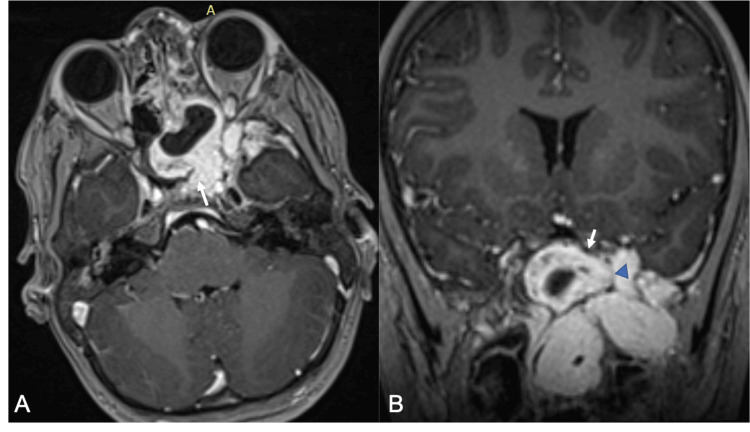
Post-contrast imaging highlighting superior extension with erosion of the sphenoid sinus and intracranial extension. Axial (A) and coronal (B) post gadolinium T1-weighted images showing superior tumor spread beyond the sphenoid sinus into the planum sphenoidale (arrow) with a small left extra-axial anterior temporal component (arrow head).
